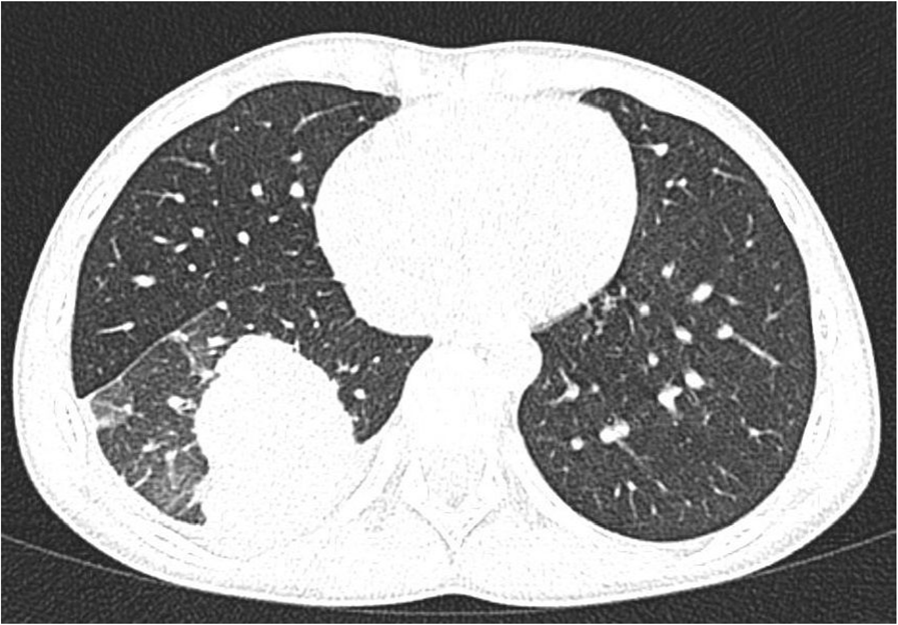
Chest CT (axial view) showing a well-defined solid mass in the right lower lobe with calcifications, surrounded by ground-glass haze and contiguous to the posterior chest wall, the diaphragm and the lung hilum
